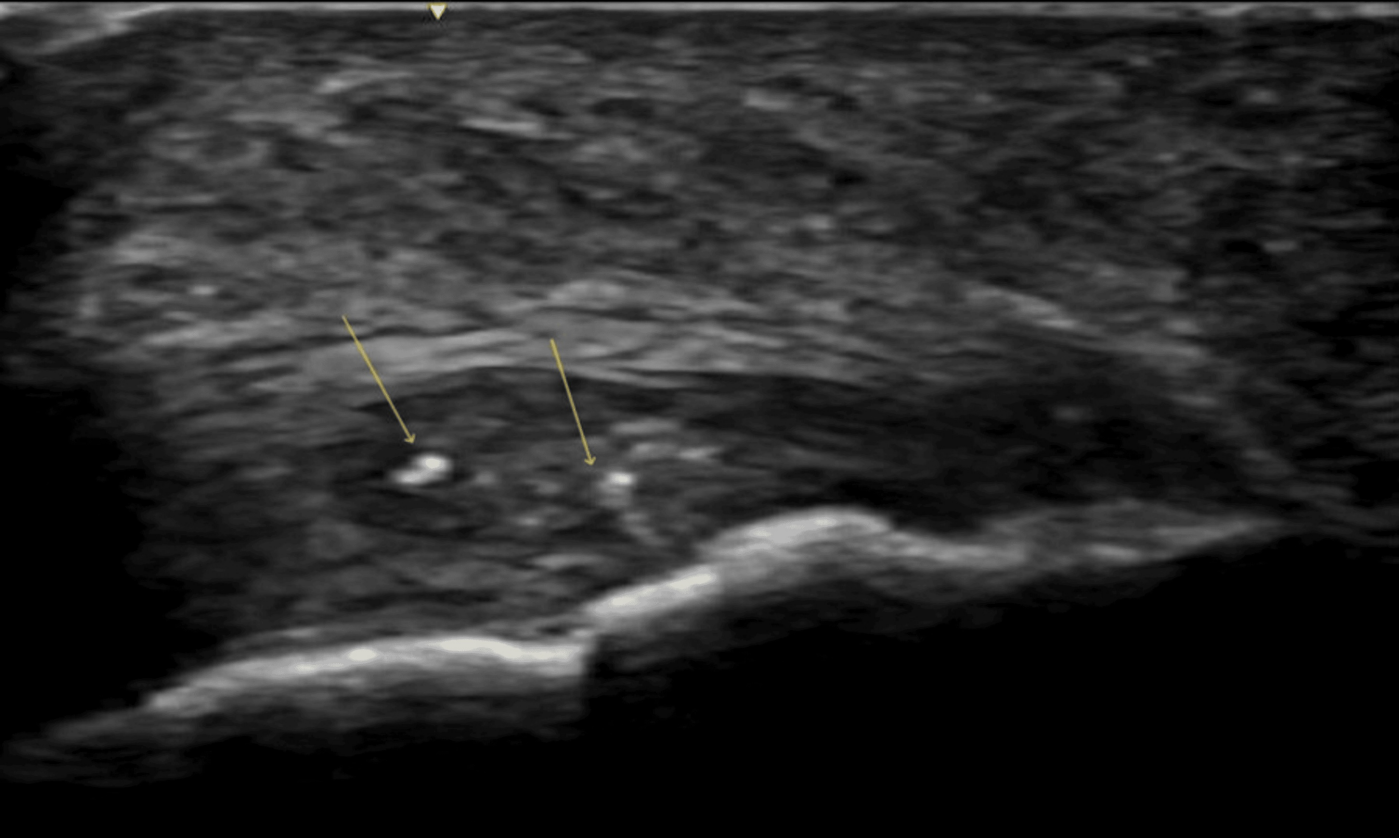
Ultrasound image of Achilles tendon in long axis Long axis ultrasound image of the Achilles tendon showing intratendinous calcifications (arrows) and alterations of echogenicity suggestive of chronic insertional tendinopathy.
